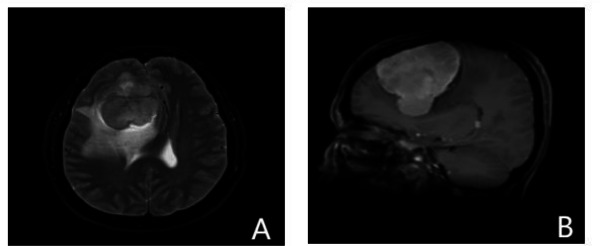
Cranial MRI: (A): horizontal view;(B): sagittal view.
